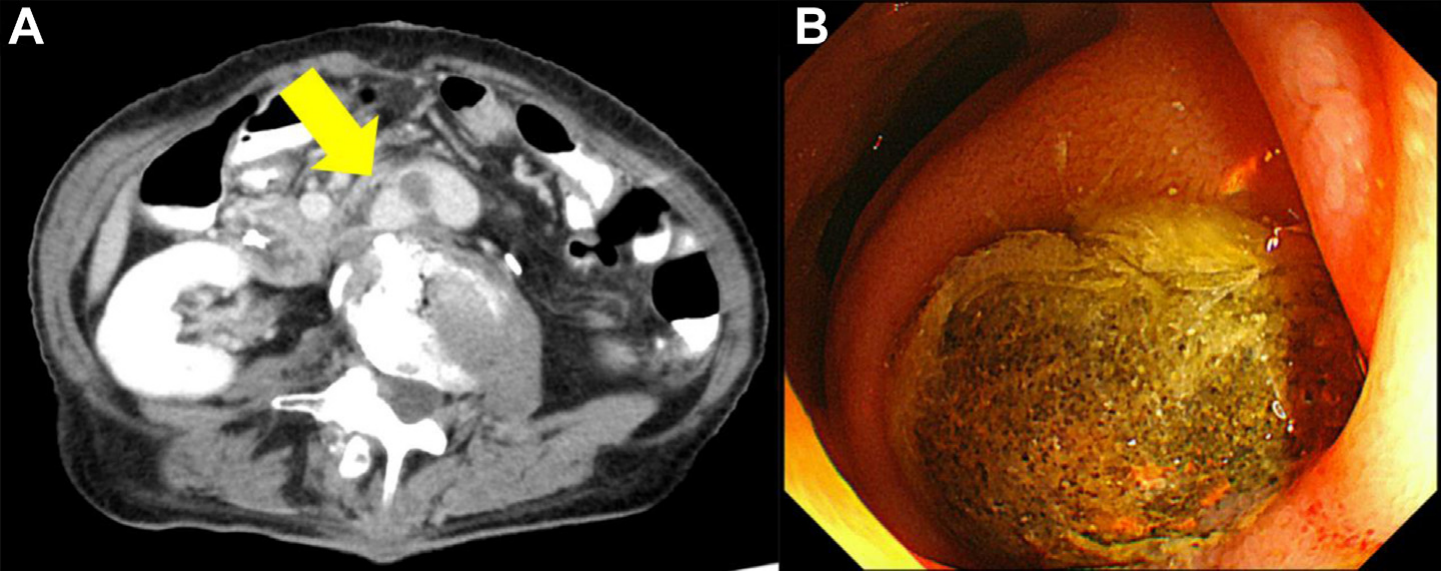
A, Preoperative computed tomography (CT) scan showing compression of the duodenum by the graft, with air bubbles around the graft (*yellow arrow*). B, Gastrointestinal fiberoptic endoscopy showing the exposed graft in the duodenal lumen.
